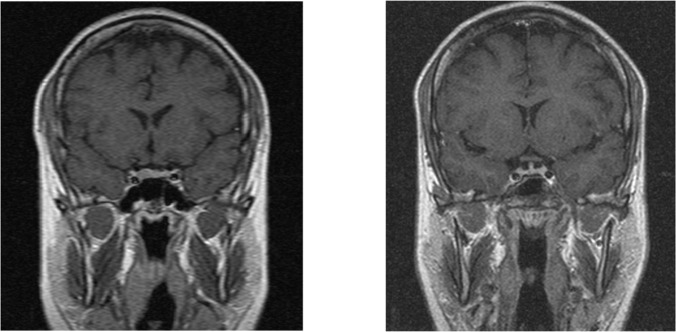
a) Coronal T1W enhanced MRI sequence through the sella turcica showing a right sellar hypoenhancing nodule suggestive of pituitary microadenoma in a patient with Cushing’s disease, b) Post-operative MRI showing no residual adenoma The patient had biochemical remission as well.
